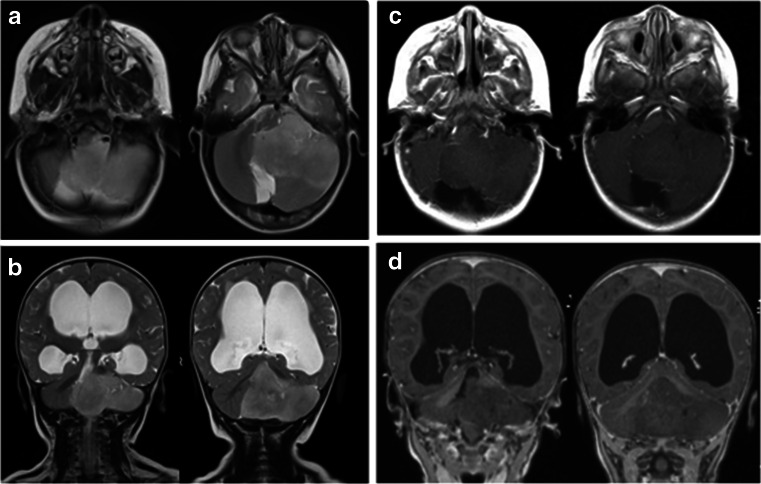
Case 2. Pre-operative MRI (a T2-weighted axial view, b T2-weighted coronal view, c post-contrast T1- weighted axial view, d post-contrast T1-weighted coronal view) showing a large CPA tanycytic ependymoma and marked hydrocephalus
